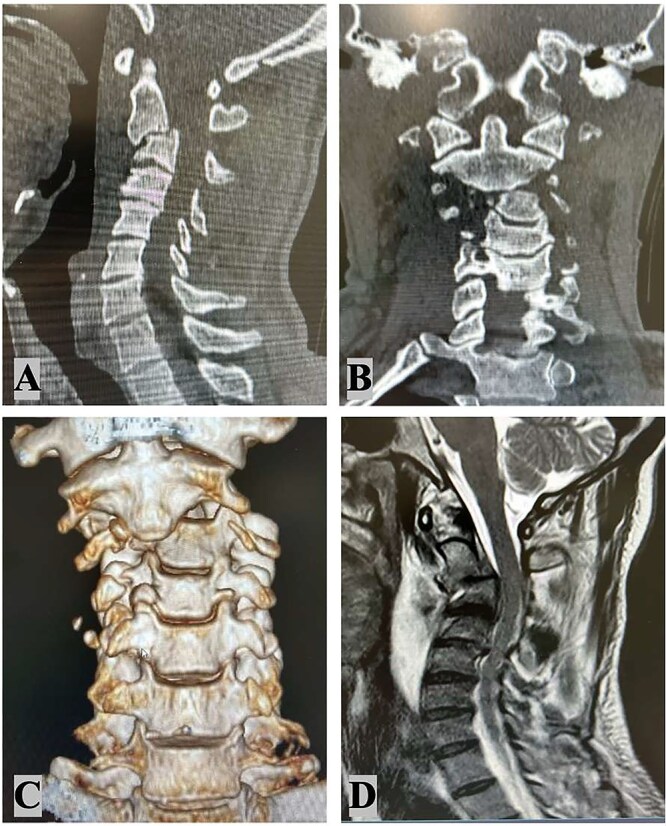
Radiological findings: Cervical spine sagittal computed tomography scan (A); cervical spine coronal CT scan (B) 3D coronal CT scan (C); MRI – C2–C3 subluxation with right lateral listhesis, where the C2 body was positioned on the right lateral aspect of C3 (D).
